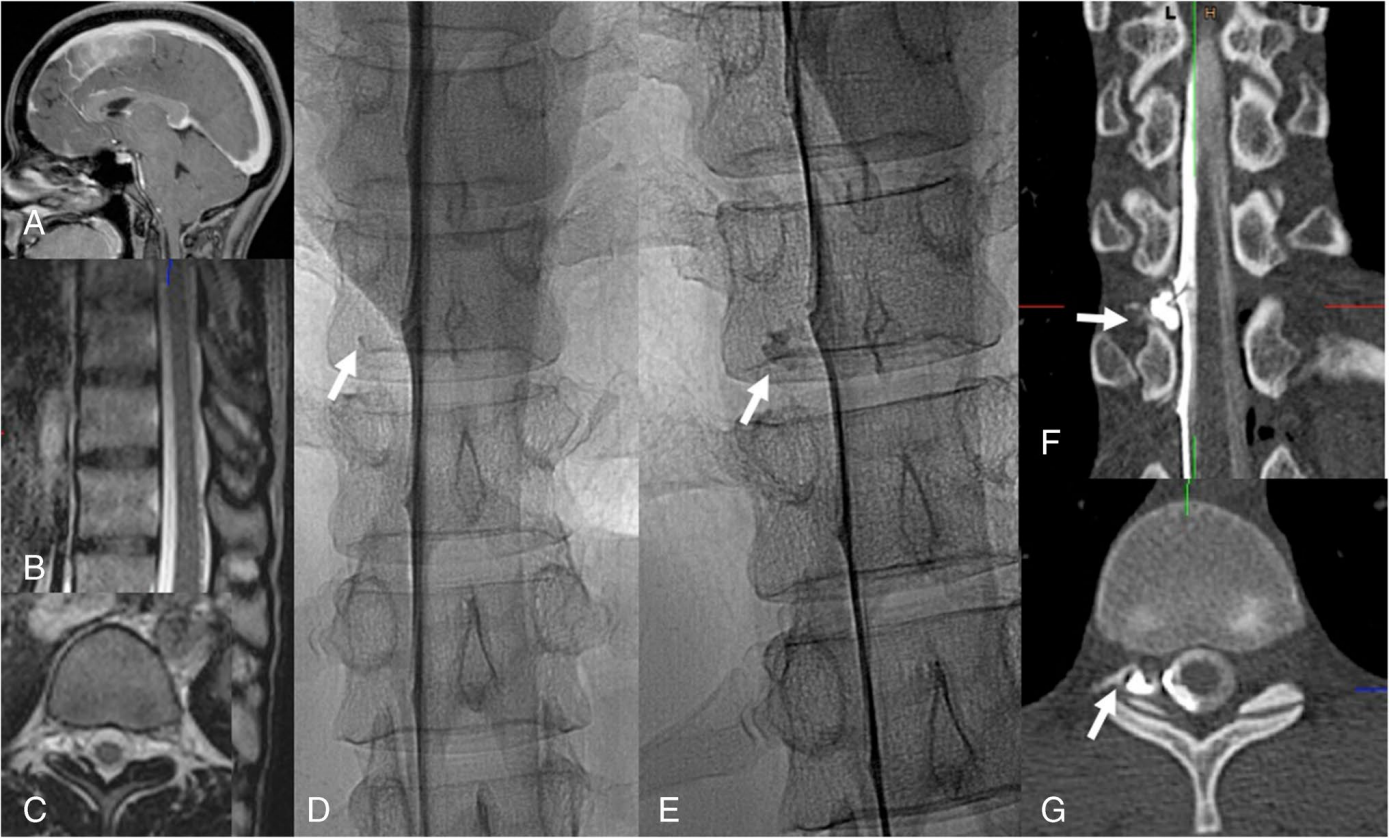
Type 2 leak according to Schievink or type 4 leak according to Farb, respectively: Head-positive (A), SLEC-negative MRI scans (B, C). Right-sided lateral decubitus dynamic myelography shows a slowly filling meningeal diverticulum (D, E: arrow). Right-sided lateral decubitus dynamic CT myelography shows the extrathecal contrast (F: G: arrow)
